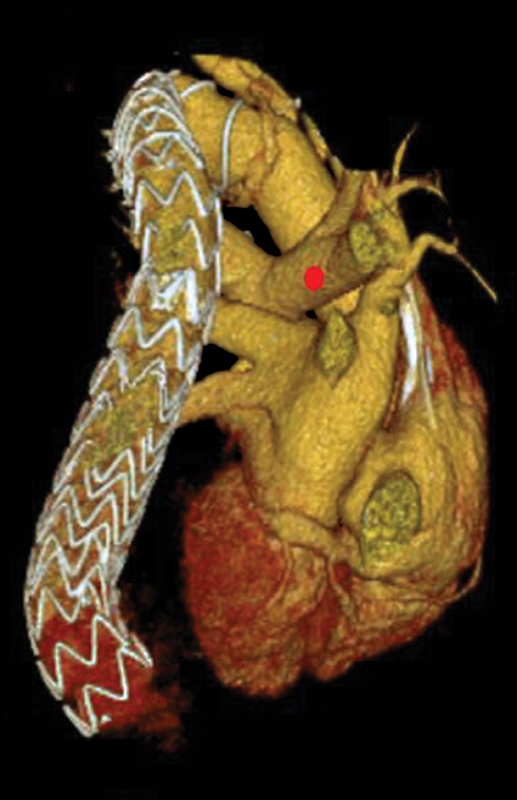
Three-dimensional computed tomography reconstruction showing Thoraflex (Vascutek Terumo) hybrid stented graft and the Valiant thoracic stent in the descending aorta. Postoperative right pulmonary artery relief (red dot).
